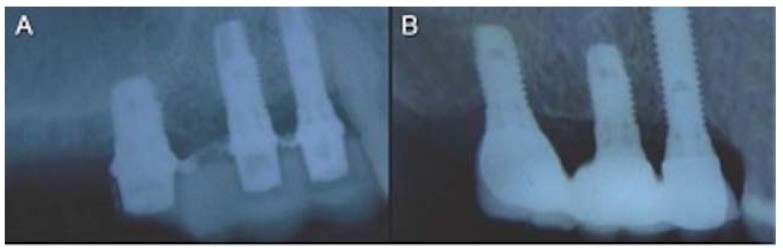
Radiographs of an immediate mixed bridge case. A) Intraoral radiographs of the provisional acrylic resin bridge. B) Intraoral radiographs of definitive mixed bridge after a mean period of 47.72 months of follow-up.
